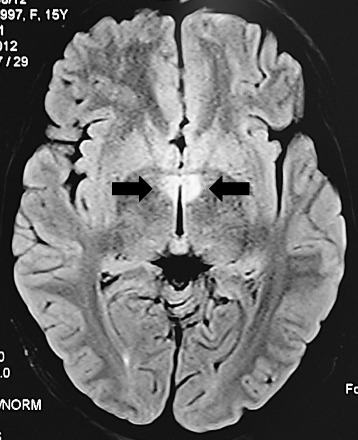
T2-weighted fluid attenuated inversion recovery (FLAIR) MRI showing high signal intensity lesions in both hypothalami (arrows).
